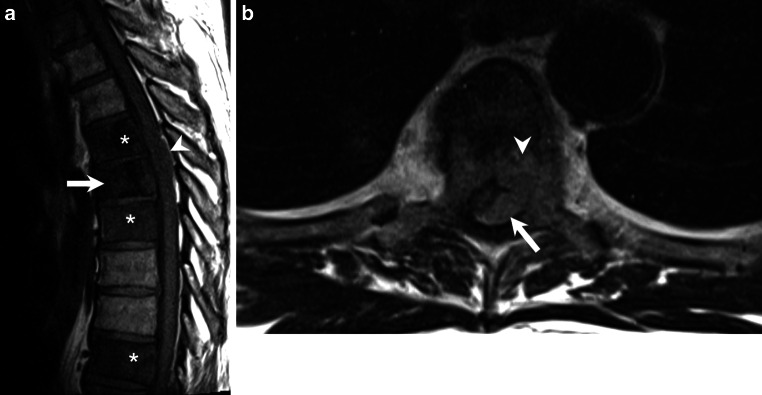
Non-enhanced sagittal T1-weighted images (a) showed decreased signal intensities in the vertebral bodies of Th5 (*arrow*), as well as Th4, Th6, and Th9 (*asterisks*). The intraspinal, perimedullary lesion clearly visible on T2-weighted imaging was hardly perceptible on sagittal native T1-weighted images (*arrowhead*). On non-enhanced axial T1-weighted images (b) at the level of Th5 the lesion appeared slightly hyperintense and seemed to be located in the epidural space (*arrow*) and showed infiltration of the vertebral body (*arrowhead*)
